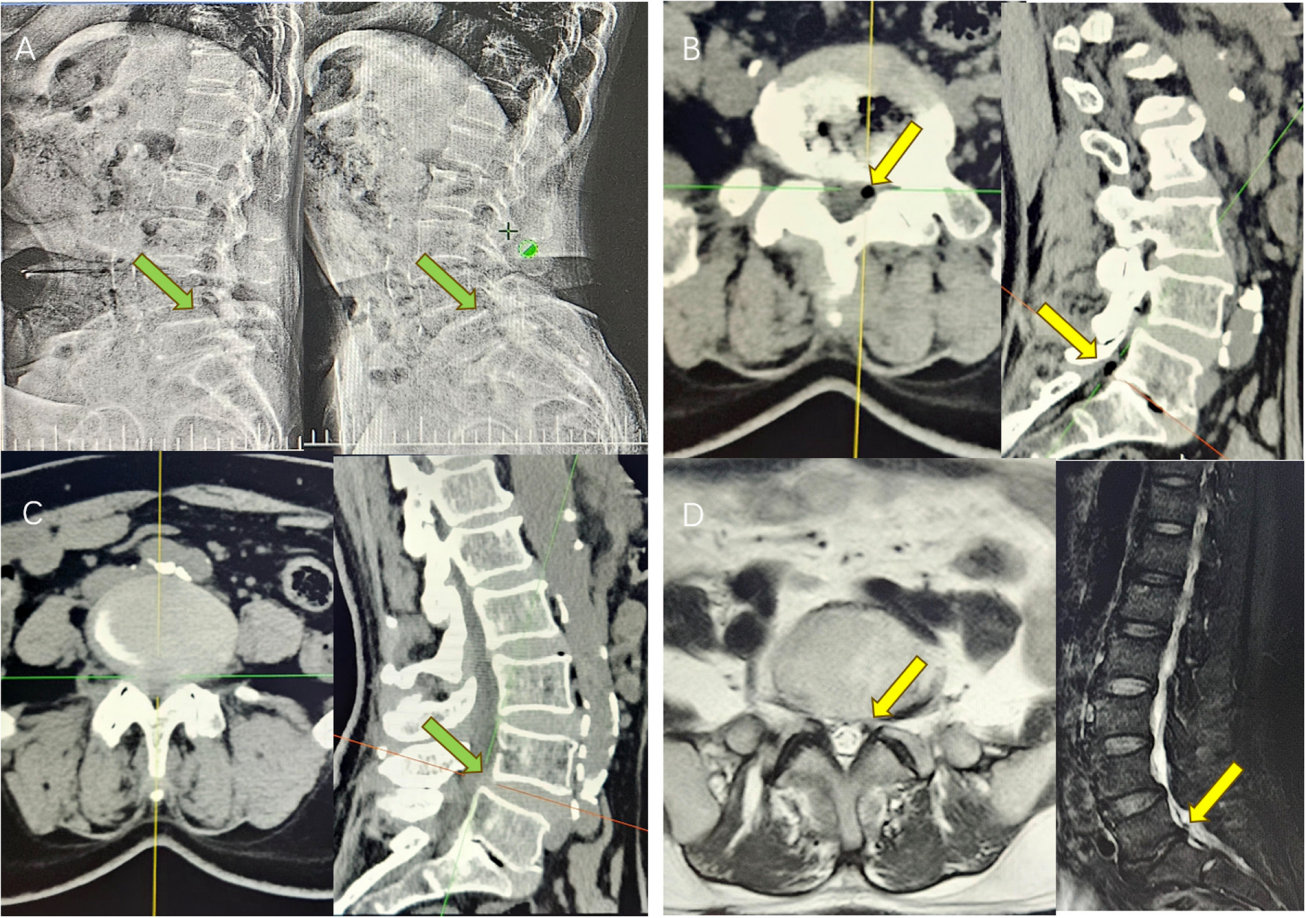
(A) Preoperative dynamic DR showed that the intervertebral height of L5/S1 segment was reduced, and L4 slipped forward I° (green arrow); (B) preoperative CT suggested that the left side of L5/S1 contained a pneumatic cyst, with the corresponding nerve root compression (yellow arrow). The intervertebral height of the corresponding segment was reduced, with the sign of intervertebral disc vacuum; (C) Preoperative CT showed that the L4 slipped forward I°, with spinal stenosis at the corresponding level (green arrow); (D) Preoperative MRI showed hypointense T1/T2 images on the left side of L5/S1 (yellow arrow).
